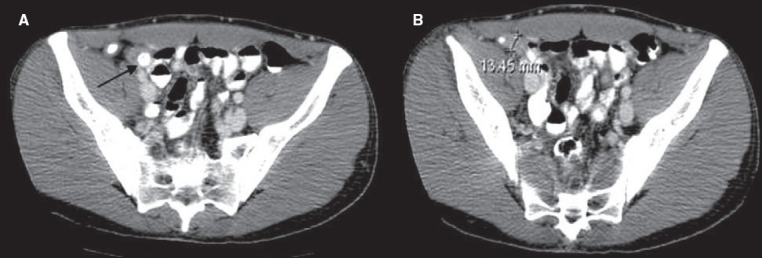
Appendicitis. Contrast-enhanced CT scan shows a dilated tubular structure in the right lower quadrant with appendicoliths (arrow)
